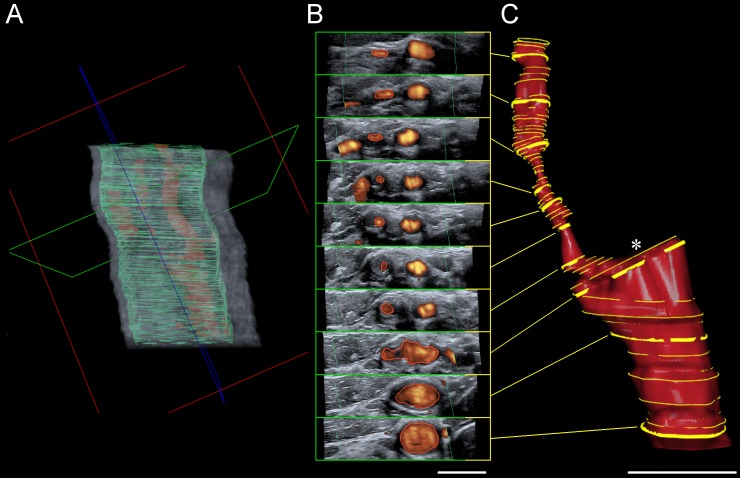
Three-dimensional ultrasound (3DUS) vessel reconstruction. (A) Two-dimensional power mode images were grabbed from the video port of the ultrasound system and concatenated with spatial and temporal information to be stored in a virtual 3D-volume. Within this virtual 3D-volume navigation in all 3 orthogonal spatial planes is possible ad libitum. Plane orientation: green = transversal, red = coronal, blue = sagittal. (B) Based on the transversal planes (green), the lumen of the common and internal carotid artery was traced manually at variable distances of 1 to 4 mm with smaller (1 mm) intersection intervals at level of the stenosis. This manual segmentation (all yellow lines) was followed by an automatic vessel reconstruction (C). The reconstructed carotid artery can be rotated freely and cross-sectional area can be measured at every point perpendicular to the vessel’s course. Asterisk indicates origin of external carotid artery (not shown). Scale bar each 1 cm.
